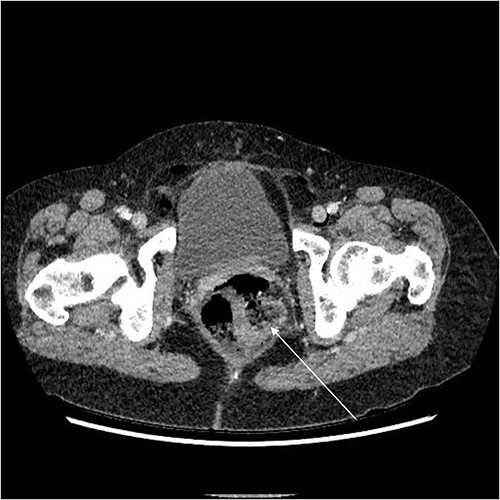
Axial CT showing rectal perforation (arrow).
